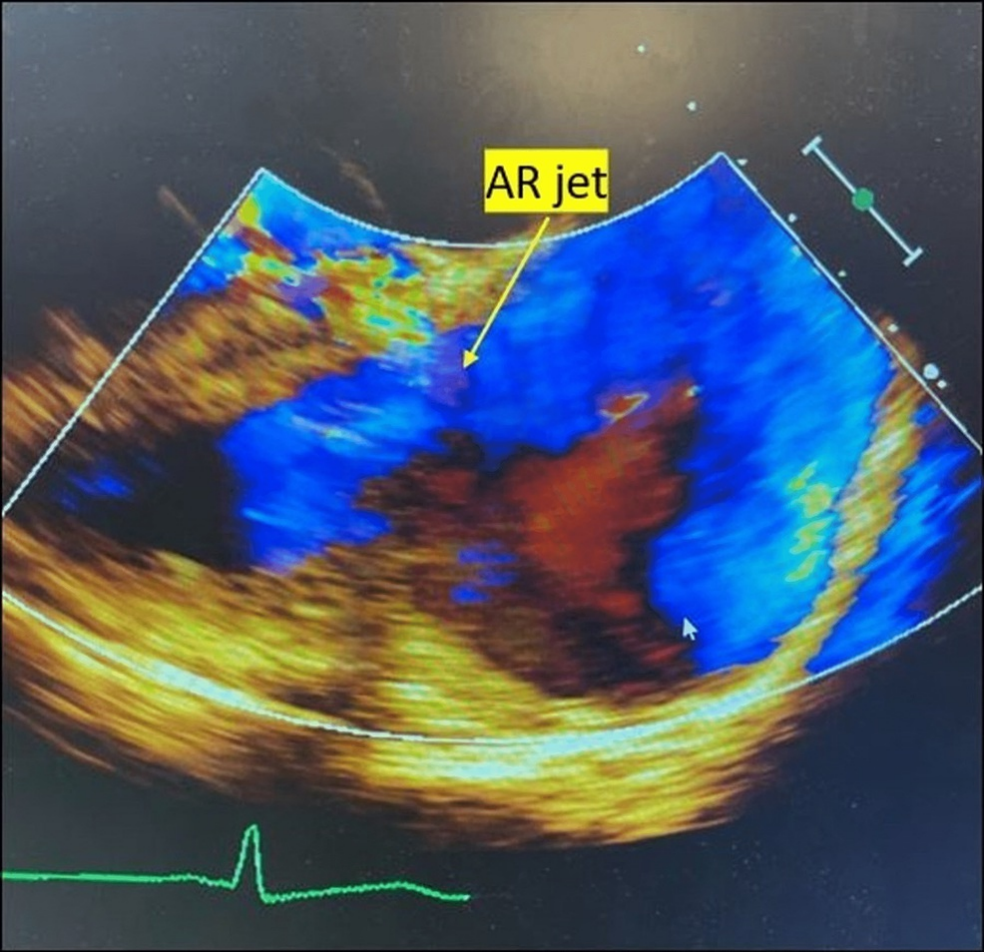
Transthoracic echocardiography showing aortic insufficiency. The echocardiogram in the parasternal long-axis view shows aortic insufficiency and aortic regurgitation (AR) jet (yellow arrow), the jet is noted to fill the left ventricular outflow tract. The flow toward the transducer is red in color, and flow away from the transducer is blue in color.
